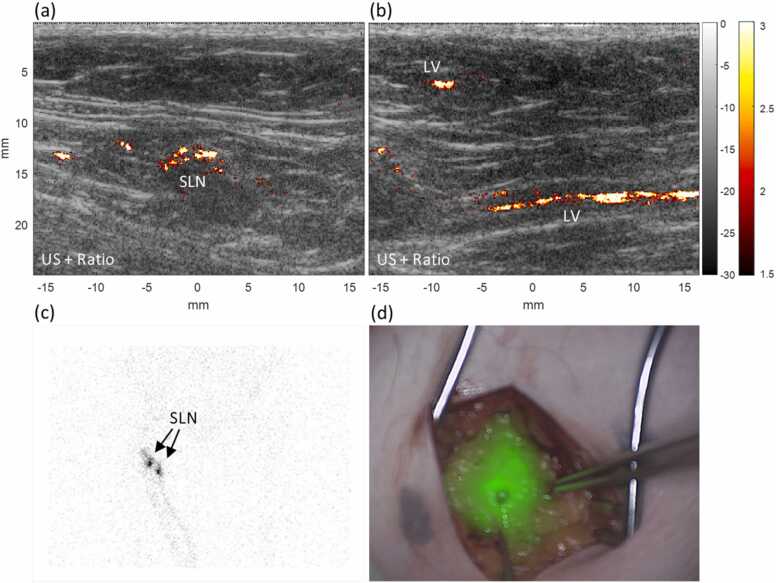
Photoacoustic imaging (PAI) results from an inguinal sentinel lymph node (SLN) and lymphatic vessel (LV) leading towards it from a patient with melanoma (patient No. 9). Ultrasound with overlaid photoacoustic ratio images of an inguinal SLN (a) and axial and parallel cross-sections of lymphatic vessels (b). Preoperative scintigraphy (c) and intraoperative NIRF imaging (d) confirmed the PAI findings.
